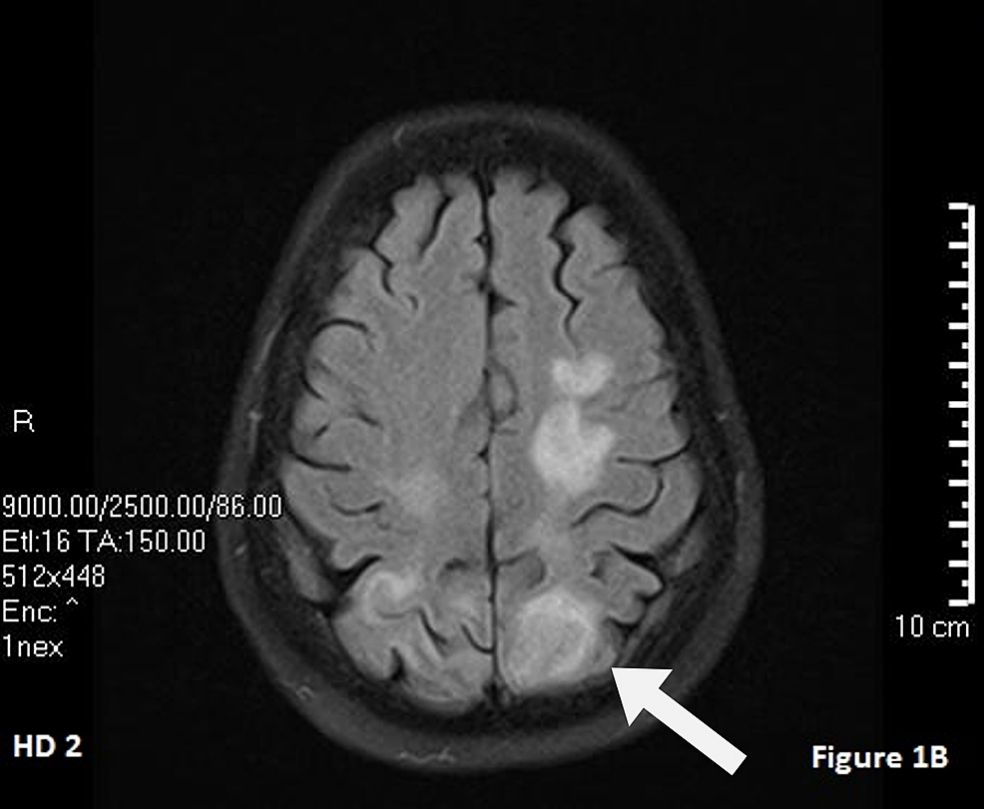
Hospital day 2 T2-flair MRI. The white arrow points to cerebral edema.
